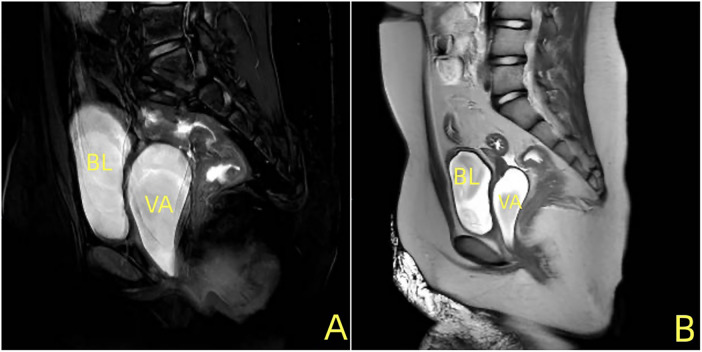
Sagittal magnetic resonance imaging (MRI) shows the presence of a fluid signal with homogeneous density in the bladder and vagina.
